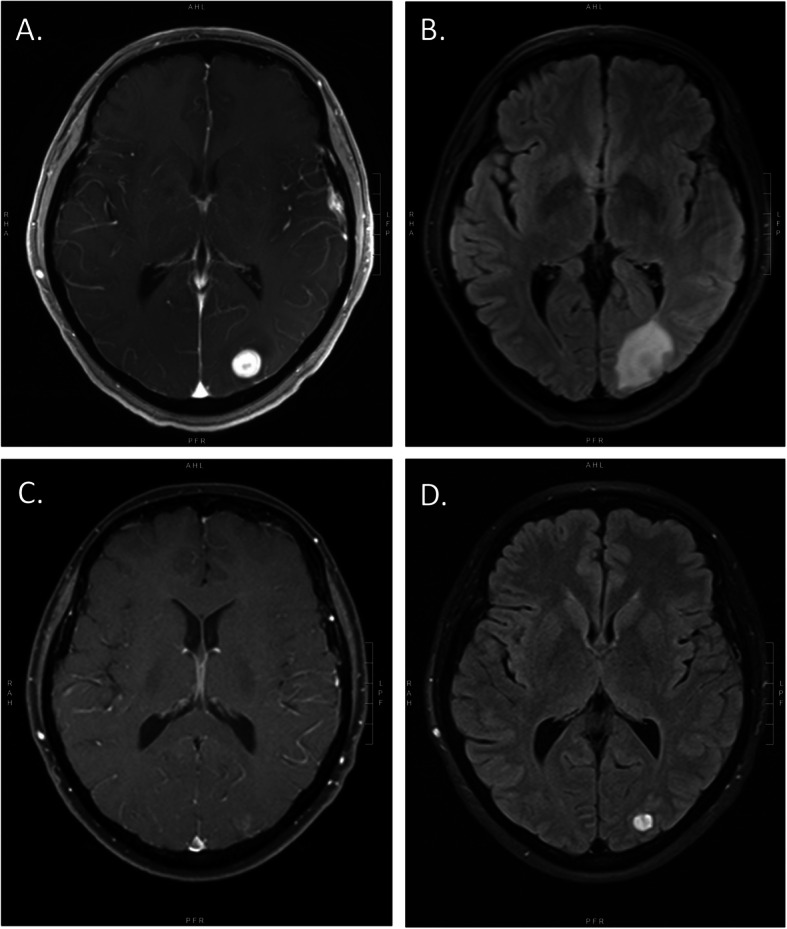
Brain MRI of a representative study participant at baseline, showing axial, T1-weighted, post-gadolinium (a) and T2-FLAIR (b) images. Brain MRI of the same participant after 10 months of protocol therapy, showing axial, T1-weighted, post-gadolinium (c) and T2-FLAIR (d) images, consistent with response to treatment
